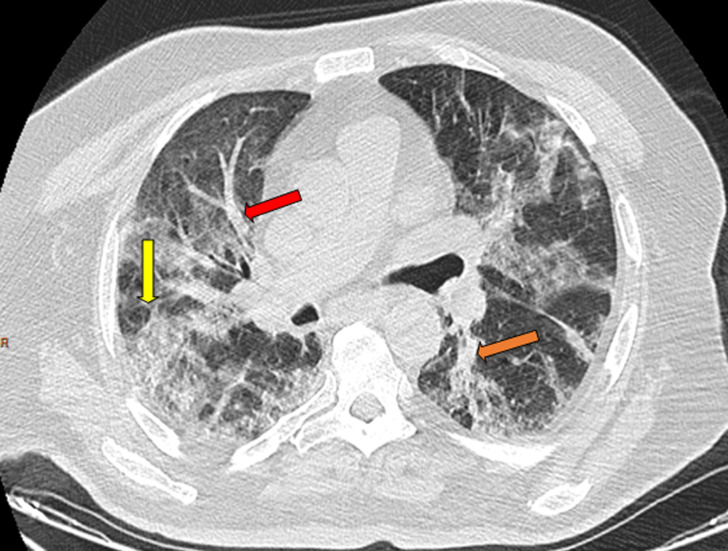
the first thoracic CT scan in an axial lung window showing widespread bilateral ground glass opacities associated with crazy paving (yellow arrow), vascular dilatation (red arrow) as well as consolidation (orange arrow)
